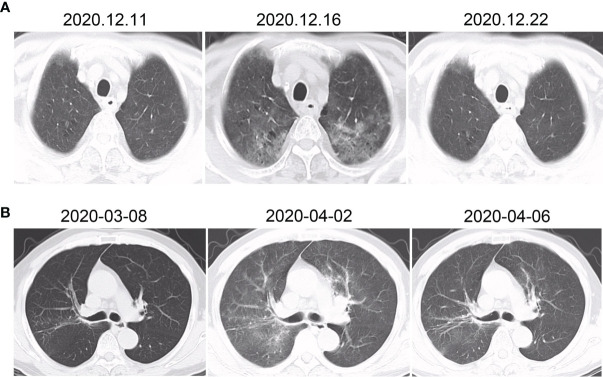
CT changes of the two patients who developed pneumonitis during the course of radiotherapy (both grade 2). The first patient (A) developed fever and cough after 23 fractions (50.6 Gy total dose), with no abnormalities initially (left). Five days later, chest CT showed ground-glass changes (center). One week after methylprednisolone commenced, the inflammation had substantially dissipated (right). The second patient (B) developed fever after two fractions (9.6 Gy total dose). Initially there were no major findings (left), but patchy infiltrates were soon found in the radiation field (center). After 4 days of methylprednisolone, the findings had significantly reduced.
